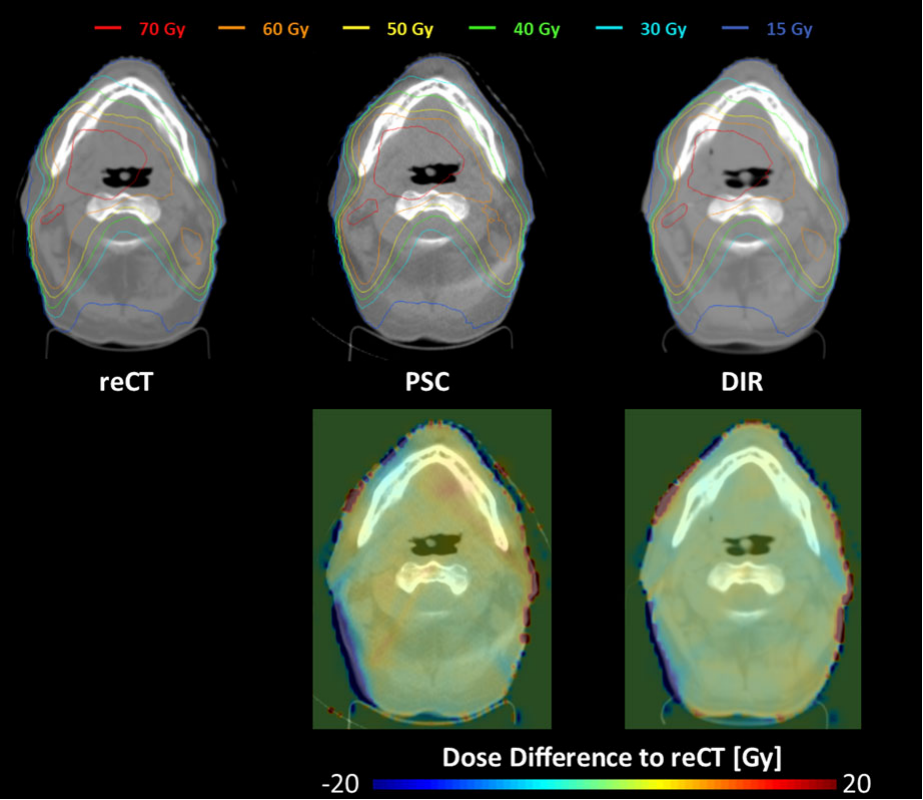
(Upper) Sample dose distributions from patient 1 for the plans calculated on the gold standard reCT (left), PSC calibrated CBCT (middle), and DIR calibrated CBCT (right) image sets. (Lower) Differences between the PSC, DIR calculated dose distribution and the reCT calculated dose distribution.
